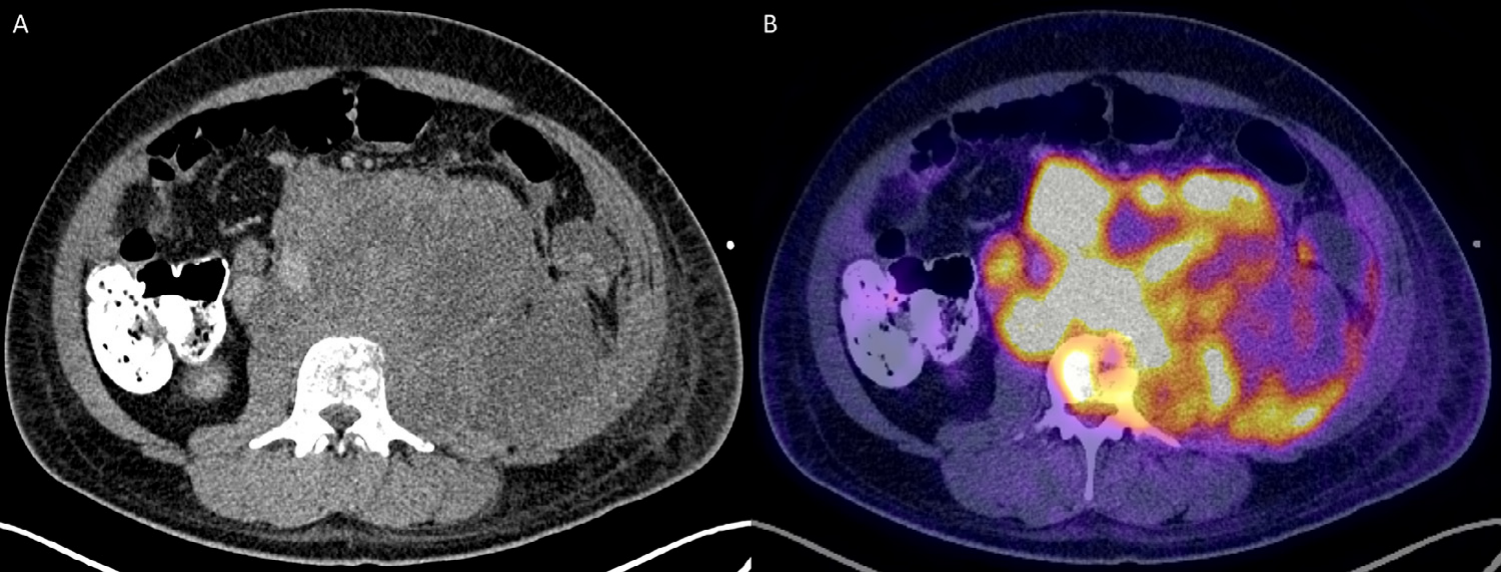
Positron emission tomography (PET) and/or computed tomography (CT) was subsequently performed. This mass exhibits peripheral, heterogenous increased FDG uptake, with extension into L1 through S1 vertebral bodies (not included in the figure).
